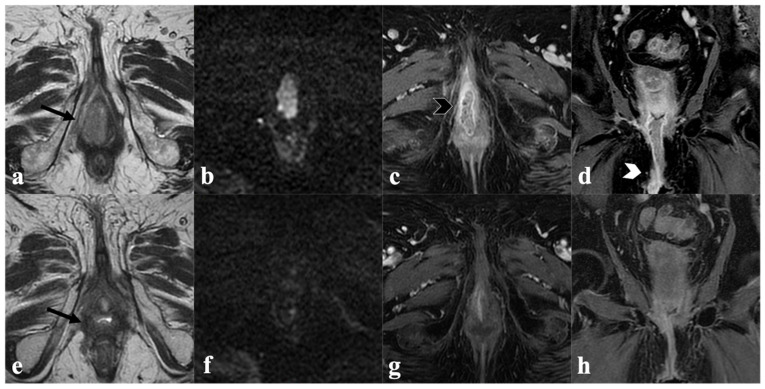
Complete response to CRT. An 85-year-old patient with locally advanced vulvar cancer (FIGO stage IIIA) treated with CRT, showing complete response to treatment. Baseline MRI (upper row) with axial T2-weighted ((a)—black arrow) axial DWI (b) post-contrast fat-saturated axial (c) and coronal (d) T1-weighted images shows infiltrative vulvar tumor involving, anteriorly, the clitoris and urethral meatus (black arrowhead in (c)), posteriorly, the fourchette, and cranially, the lower third of vaginal introitus (white arrowhead in (d)). MRI after CRT (bottom row) shows absence of a T2 intermediate signal intensity mass ((e)—black arrow), no hyperintensity in DWI (f), and no enhancement in post-contrast images (g,h) consistent with complete response.
